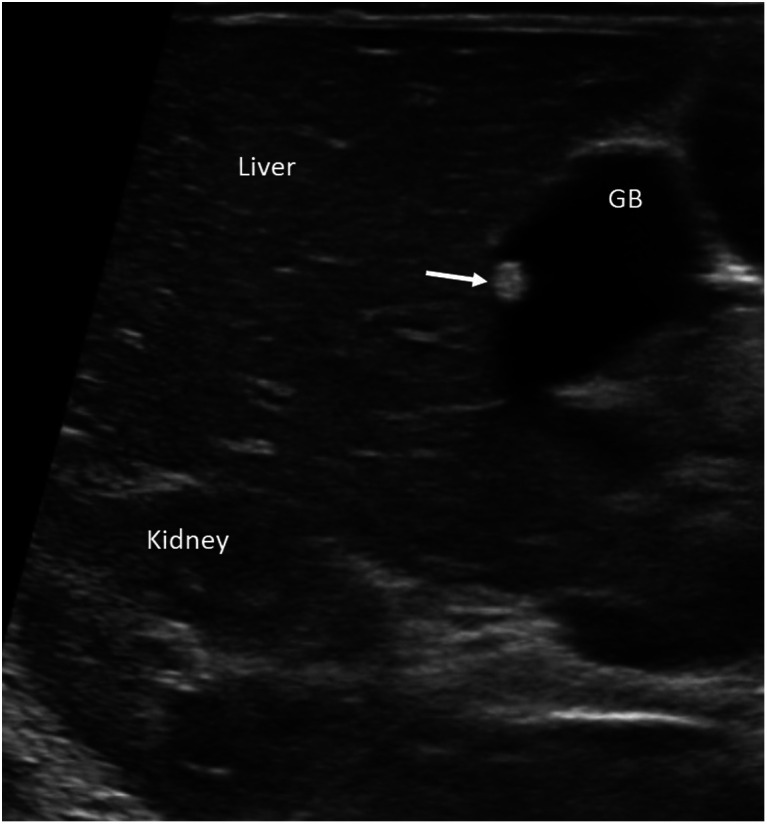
Transverse abdominal US of the gallbladder (case number 8) shows an echogenic polyp *(arrow)* in the gallbladder. GB, gallbladder; US, ultrasonography.
